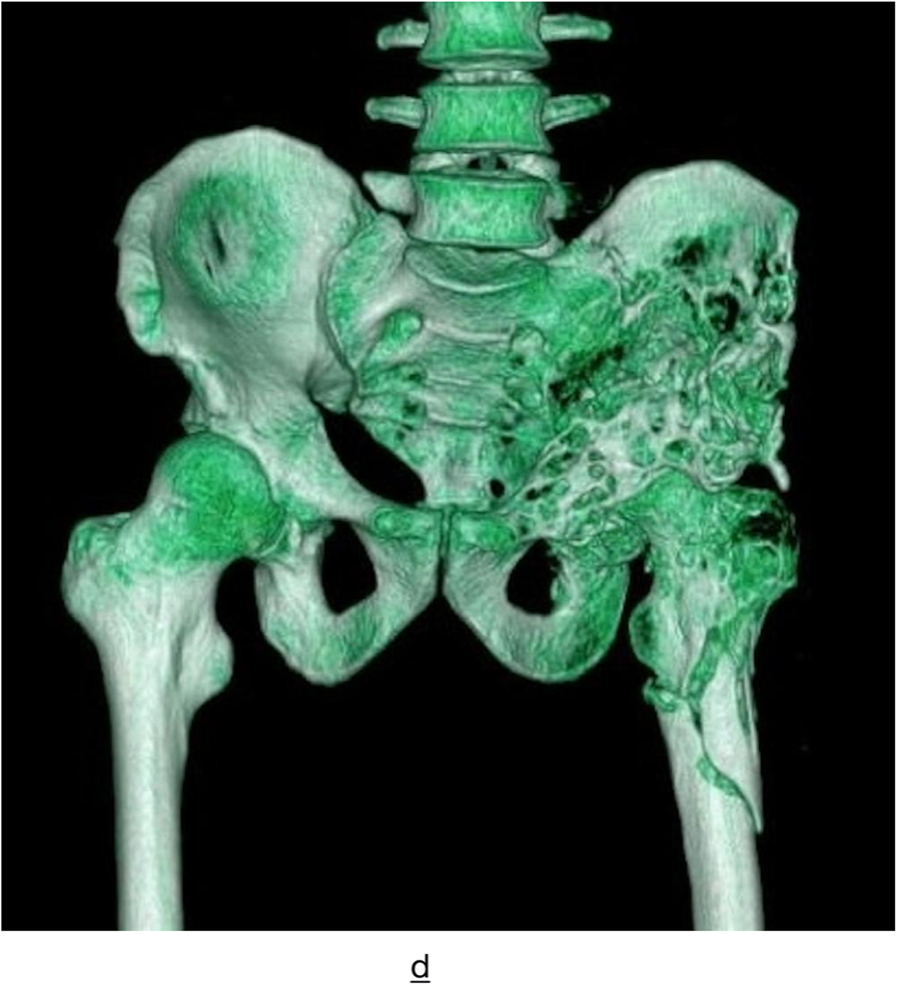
CT 3D reconstruction showing multiple wormlike bone destruction in the left margin of the sacrum, left ilium, pubis, and part of ischium, acetabulum, and upper femur, and the continuity of bone cortex of the left proximal femur was interrupted
